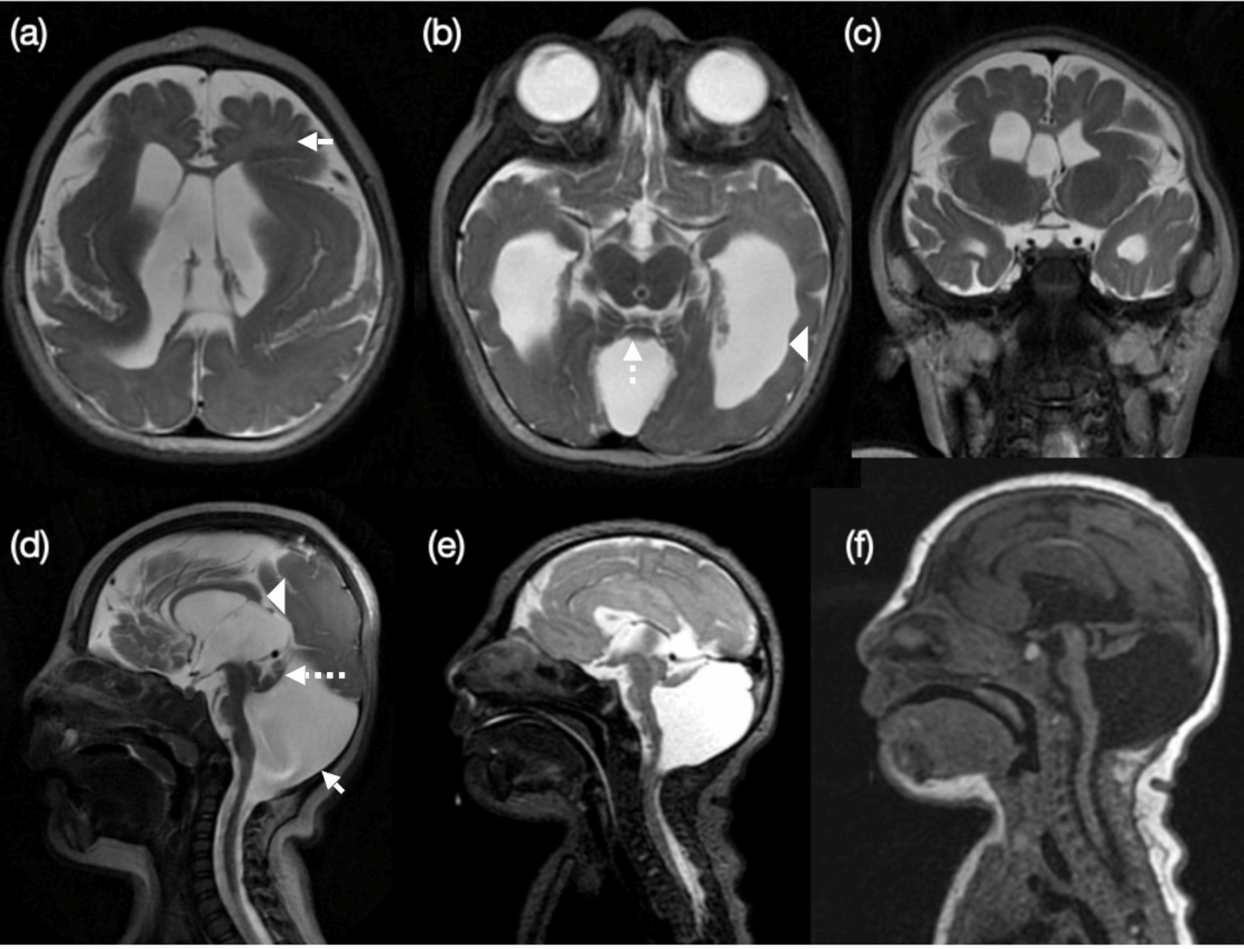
MRI brain (axial, coronal, sagittal) showing cystic dilatation of the fourth ventricle with enlarged posterior fossa (d, solid arrow) and hypoplastic, cephalad-rotated vermis (d, dashed arrow). Findings include severe microcephaly (the craniofacial index is severely low for age) with global white matter loss (a, solid arrow), simplified gyral pattern, colpocephalic lateral ventricles (b, arrow head) with preserved corpus callosum (d, arrow head), brainstem atrophy, and underopercularized Sylvian fissures.
